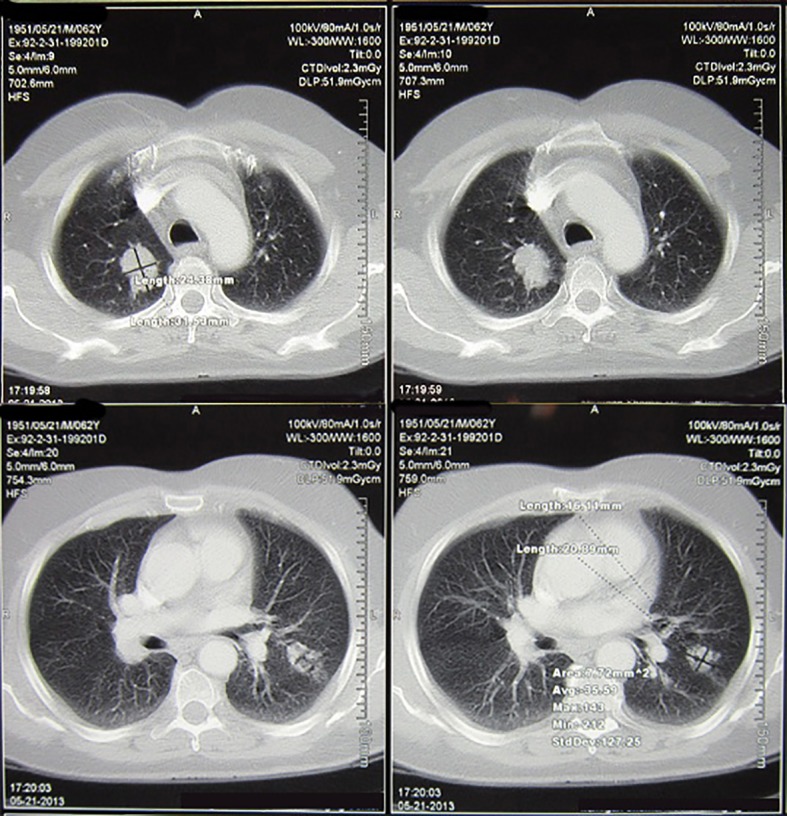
Shows the chest CT scan of the patient 3 weeks after the 8th cycle of chemotherapy with docetaxel.
